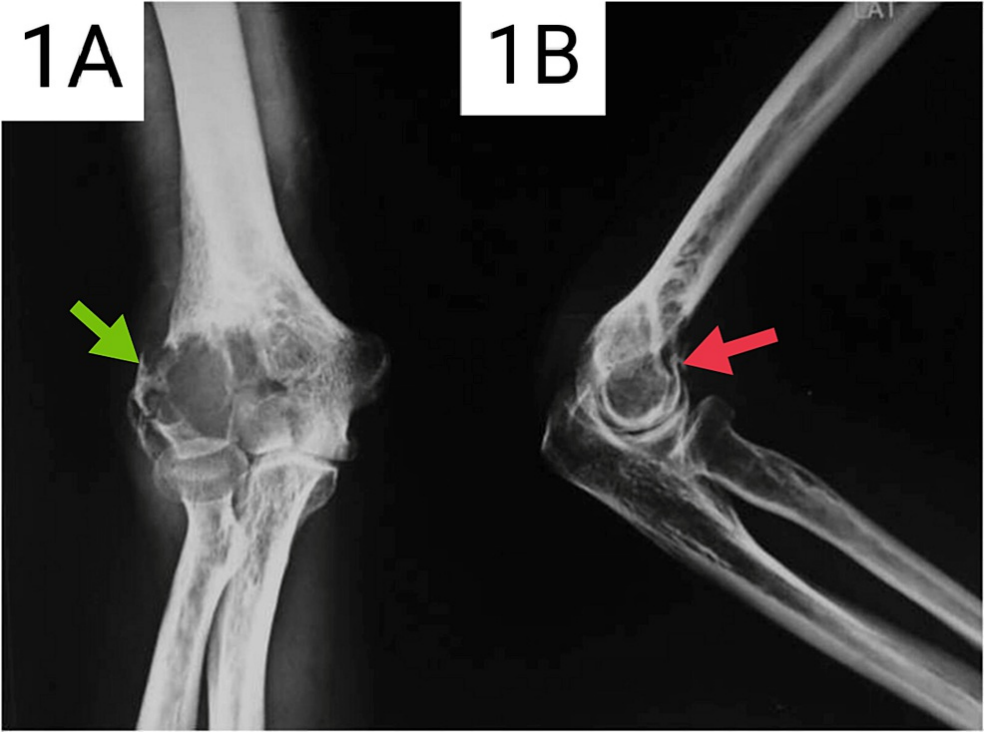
(A) Anteroposterior radiograph of the right elbow at the time of initial presentation. (B) Lateral radiograph of the right elbow at the time of presentation. Green arrow - Lytic lesion with "soap bubble" appearance. Red arrow - Lytic lesion in the distal humerus
